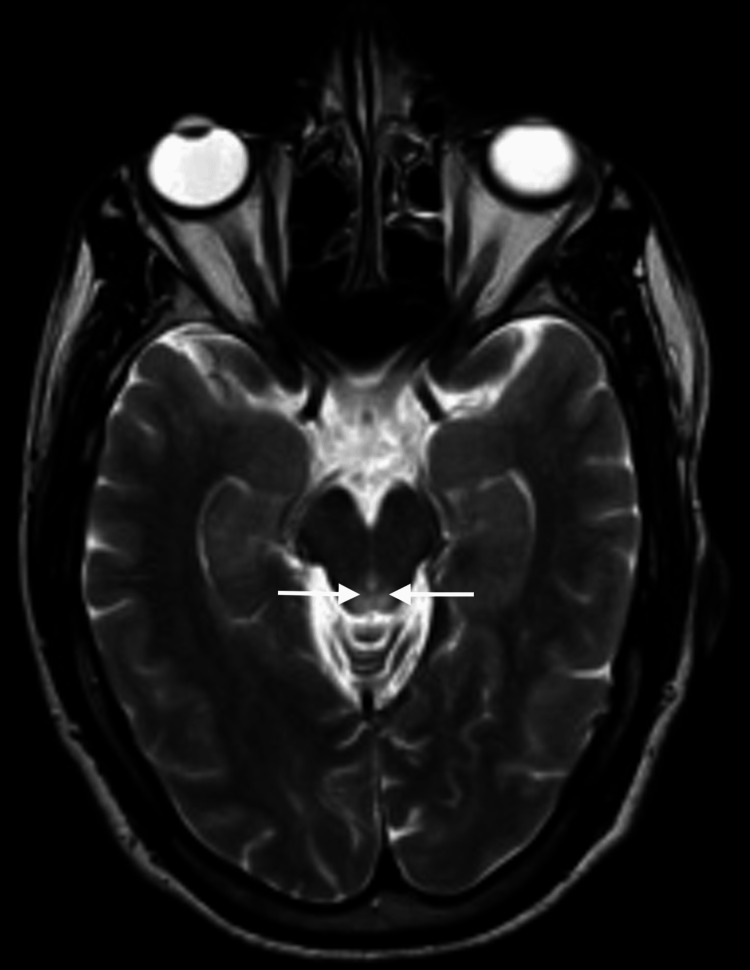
Contrast-enhanced MRI: An initial study. Axial T2. Periaqueductal bilateral and symmetrical hyperintensity around the third ventricle, in the periaqueductal gray matter, is observed on T2-weighted images (arrows).
